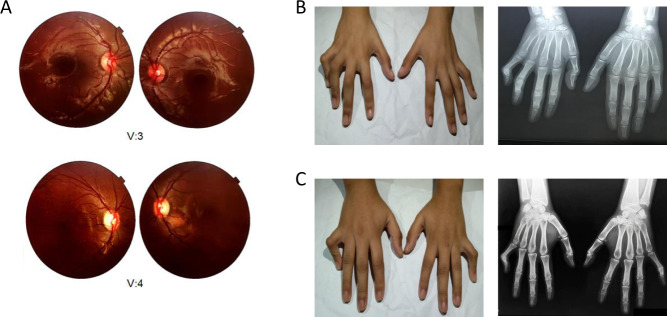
Fundoscopy and hand x-rays and photographs for two affected family members. a Fundoscopy images for V:3 (left) and V:4 (right). b Hand photograph and x-ray image for V:3 suggest clinodactyly due to shortening of middle phalanges of bilateral fifth and fourth digits resulting in radial curvature of little fingers and the fourth digit of the right hand toward the right. c Hand photograph and x-ray image for V:4 suggest clinodactyly due to shortening of middle phalanges of bilateral fifth digits resulting in radial curvature to little fingers toward the right
